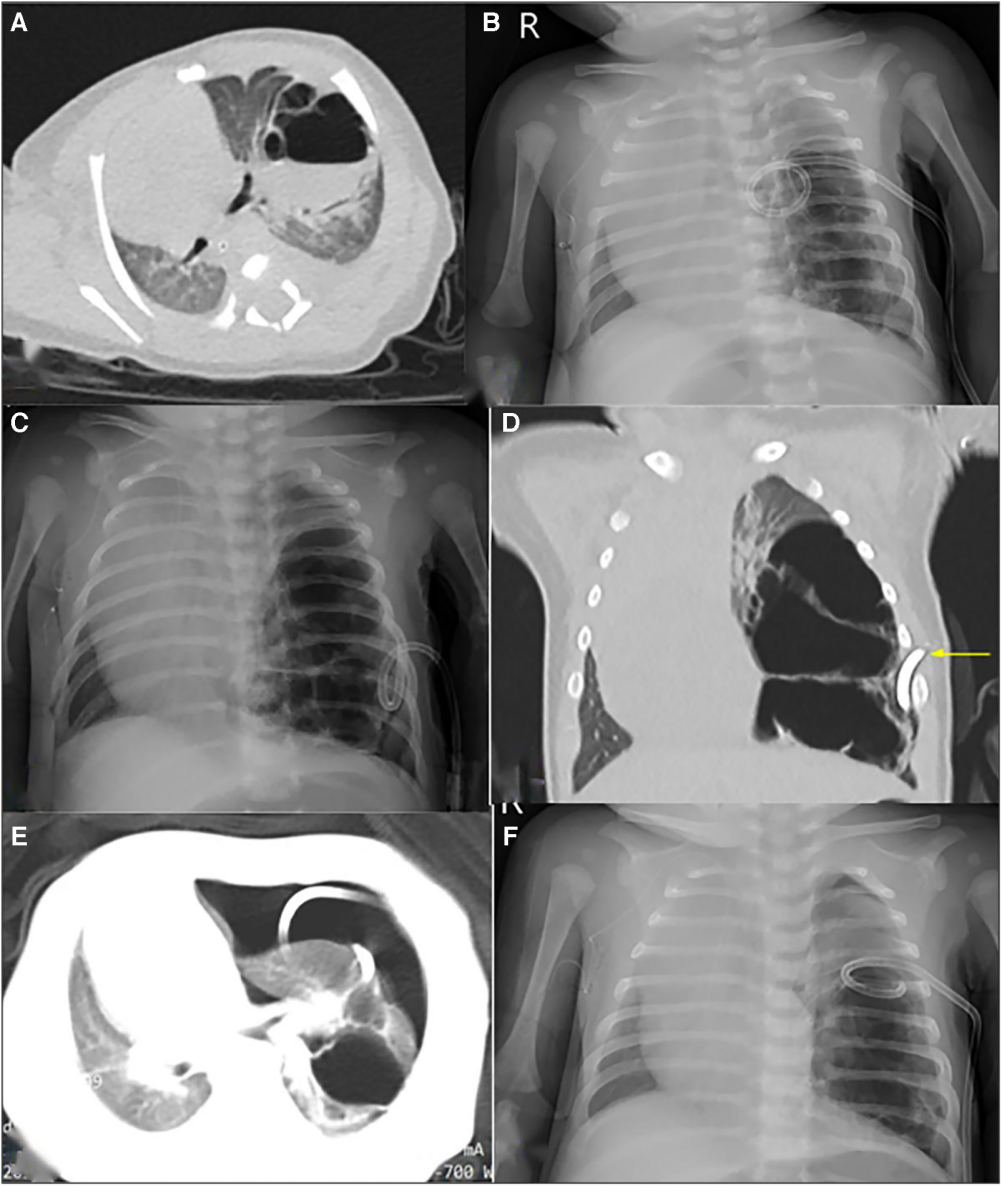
Radiological imaging of PTCD maneuvre in case 5. (A) Preoperative CT scan showed a large cystic lung lesion in the left lower lobe, during which an liquid-air level was noted; (B) and (C) The first and second post-PTCD radiological image both displayed little relief of mass effect, respectively; (D) CT scan indicated the lung lesion was multicystic and could not be completely decompressed by the pigtail catheter (yellow arrow); (E) CT scan shown pneumothorax occurred secondary to the 2nd PTCD; F: Chest X-ray indicated the air in the thoracic cavity was drained via catheter adjustment.
